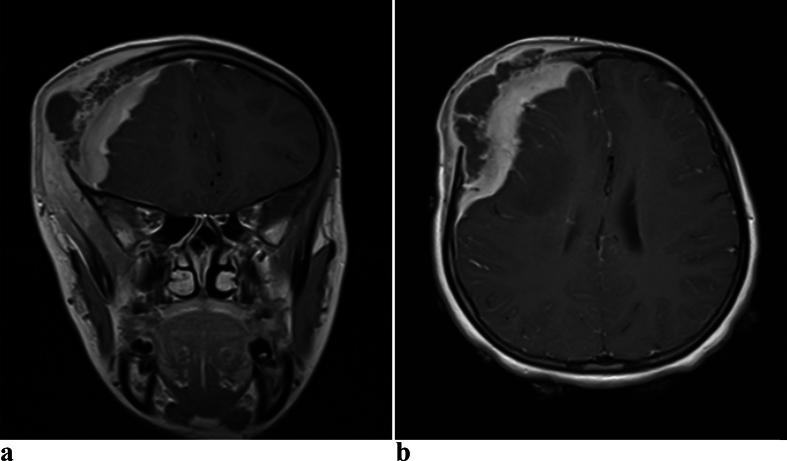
a. Contrast MRI T1W1 coronal image of brain showing extradural and subgaleal enhancing lesion with central mixed enhancing and non enhancing hypo intense area with mass effect and midline shift b. Contrast MRI T1W1 axial image of brain showing extradural and subgaleal enhancing lesion with central non enhancing hypo intense area with mass effect on the brain and lateral ventricle with midline shift.
